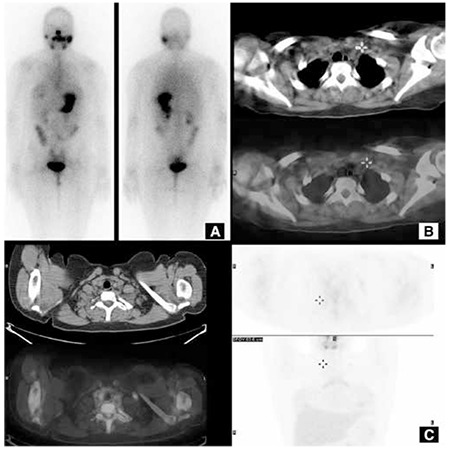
A 16-year -old female admitted to nuclear medicine department with a diagnosis of 1.7 cm papillary thyroid cancer and multiple right cervical lymph node metastases. She received 150 mCi RAI therapy. On follow-up, the thyroglobulin level (54.4 ng/mL) was elevaletd with a TSH=87μIU/mL. The I-131 wholebody (A) and SPECT/CT imagings were normal (B). F-18 FDG PET/CT showed FDG uptake (SUV_max_: 3) left supraclavicular lymph node (C)
